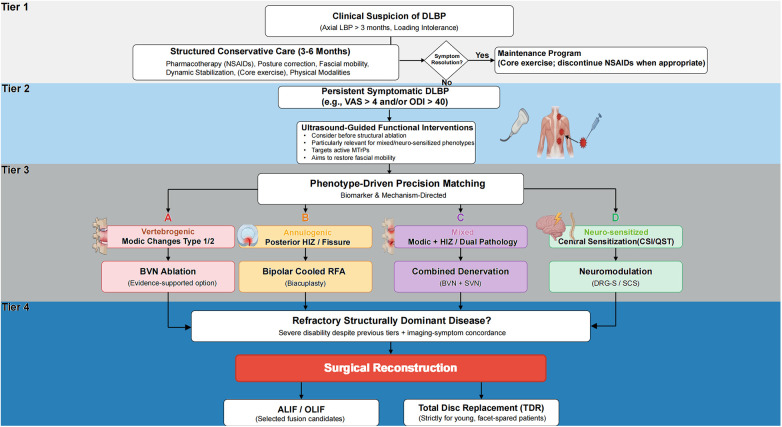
Staged phenotype-based treatment algorithm for DLBP. Tier 1 emphasizes education, pharmacological modulation, active rehabilitation, posture correction, fascial mobility restoration, core stabilization, and manual therapy. Tier 2 addresses persistent functional modifiers using ultrasound-based assessment and targeted functional interventions such as ultrasound-guided dry needling (US-DN) when concordant MTrPs, paraspinal hypertonicity, or impaired TLF gliding are identified. Tier 3 applies phenotype-specific interventions, including BVN ablation for vertebrogenic pain, biacuplasty for annulogenic pain, combined BVN/sinuvertebral nerve strategies for selected mixed cases, and neuromodulation for refractory neuro-sensitized cases. Tier 4 is reserved for surgical reconstruction, including anterior/oblique lumbar interbody fusion (ALIF/OLIF) or total disc replacement (TDR), in refractory structurally dominant cases after multidisciplinary reassessment.
